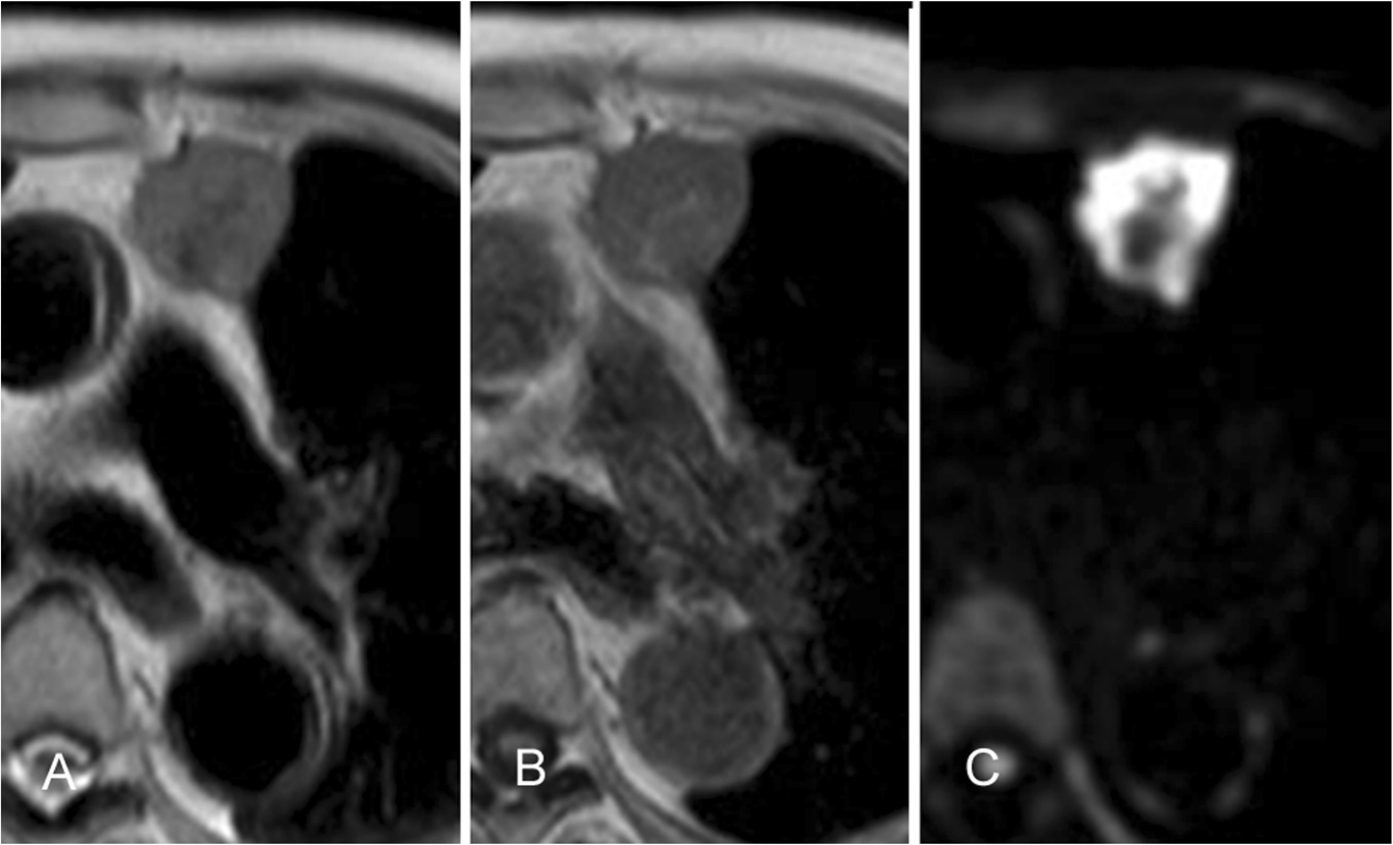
MRI findings. MRI shows the iso-intensity mass on T1-weighted imaging (a) and high intensity on T2-weighted imaging (b). The marginal zone of the mass shows a low-density area with a refined lobulated structure. Diffusion-weighted image shows a high intensity area in the marginal zone with apparent diffusion coefficient sequences (C)
